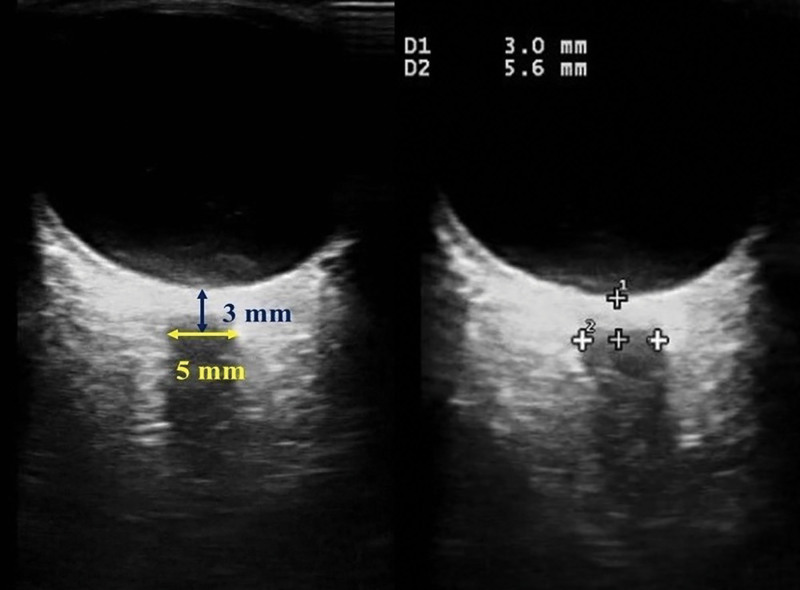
Optic nerve sheath diameter ultrasound image.
